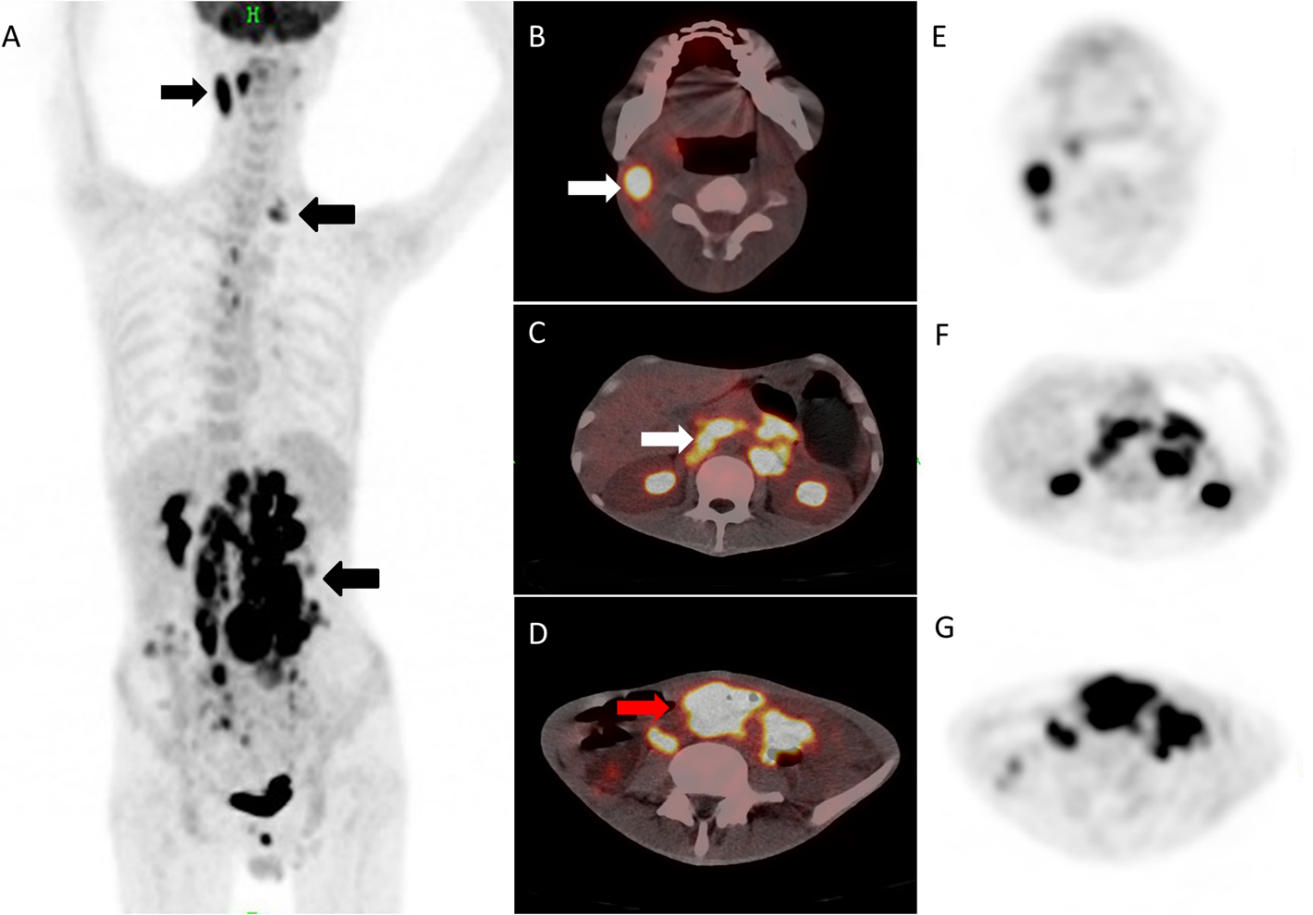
The MIP of ^18^F-FDG PET/CT (a) revealed multiple hypermetabolic lesions in the whole body (black arrows). Axial slices showed cervical, retroperitoneal and mesenteric lymph node enlargement (SUV_max_ = 11.1, white arrows) and diffusely thickened intestinal wall (SUV_max_ = 10.3, red arrow) on PET/CT fusion (b-d) and PET (e-g)
